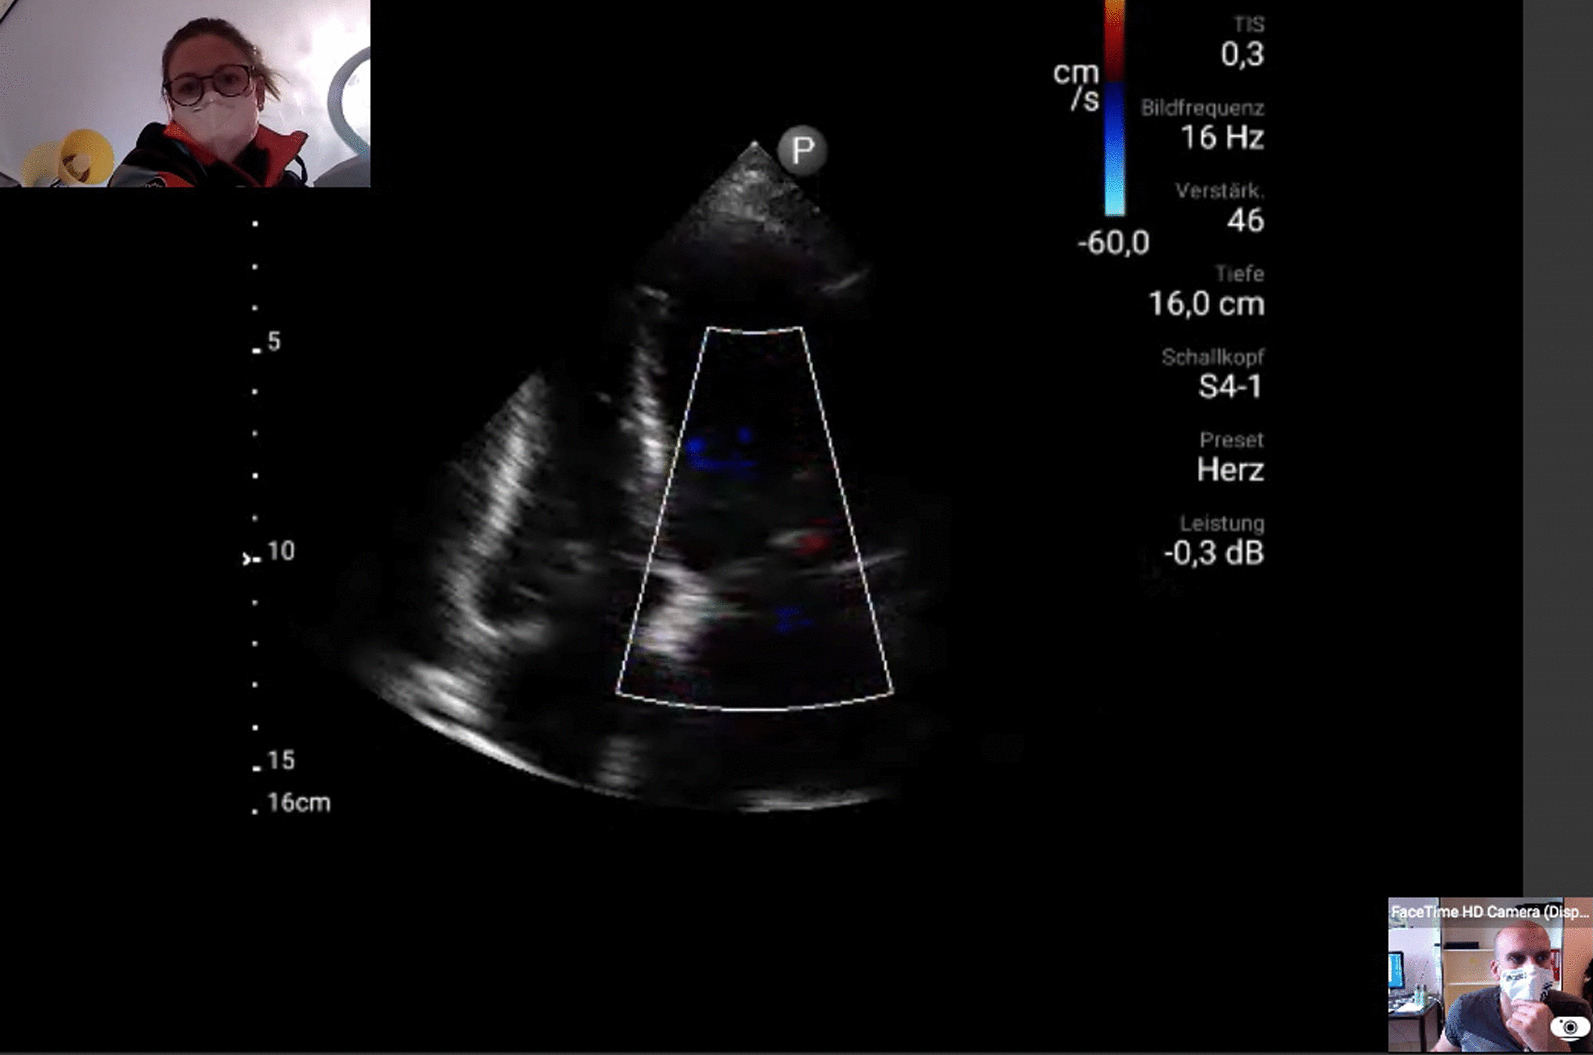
Supervisor’s view: ultrasound image, prehospital emergency doctor left corner, supervisor right corner
